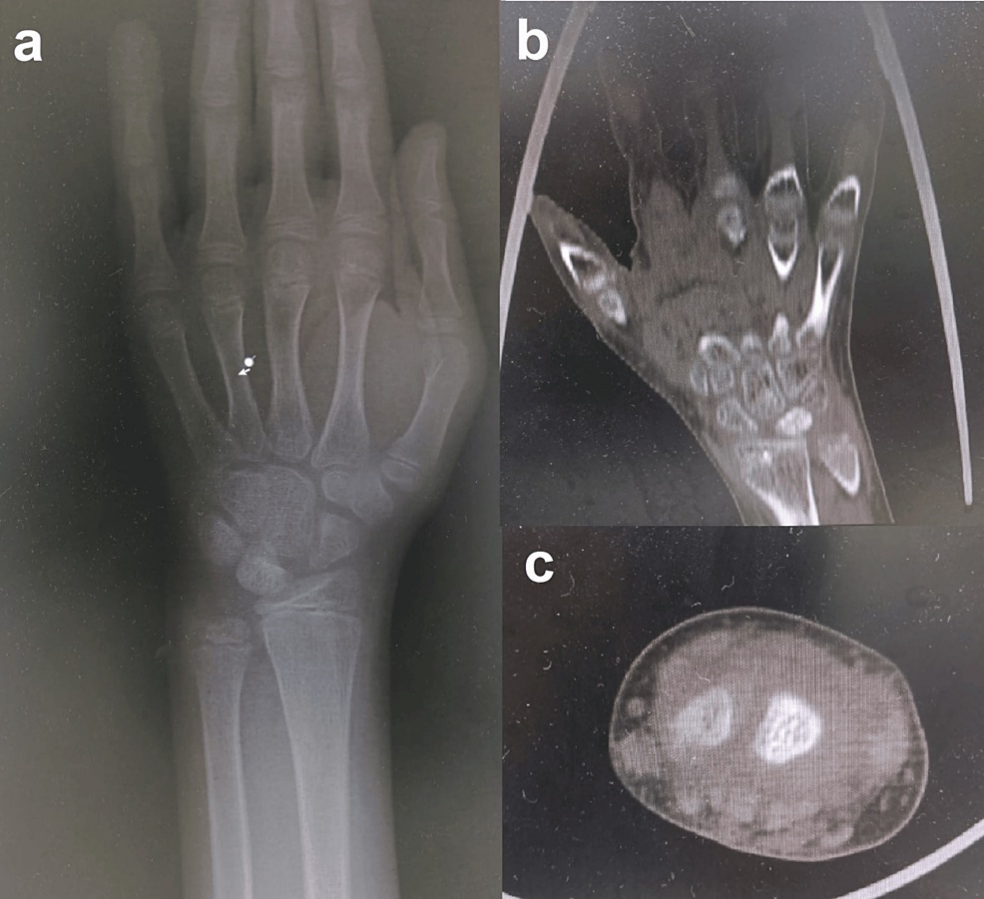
(a) Posterior-anterior left wrist radiographs demonstrated lunate bone sclerosis and widening of scapholunate space. (b, c) Coronal and axial wrist CT scans showed lunate bone sclerosis.
